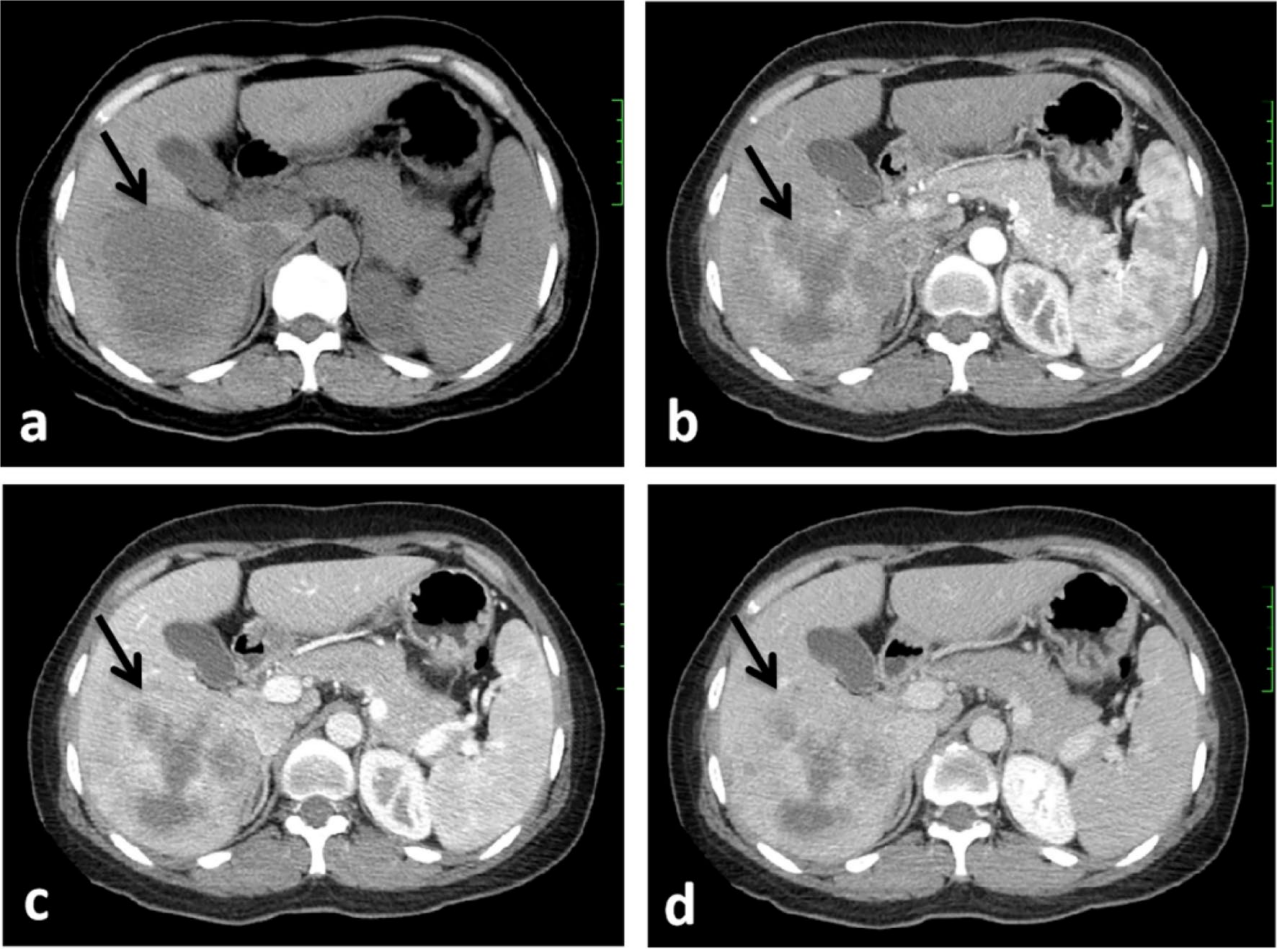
CT images in a 34-year-old HBV-positive woman with ICC confirmed by surgical resection, AFP 9.98 ng/ml, CA19-9 507.13U/ml, and its enhancement pattern was categorised as type III. Precontrast image (a) showed a solitary tumor (arrow) in the right lobe of the liver; arterial phase (b), portal phase (c) and delayed phase (d) showed rim hyperenhancement and central most necrosis.
